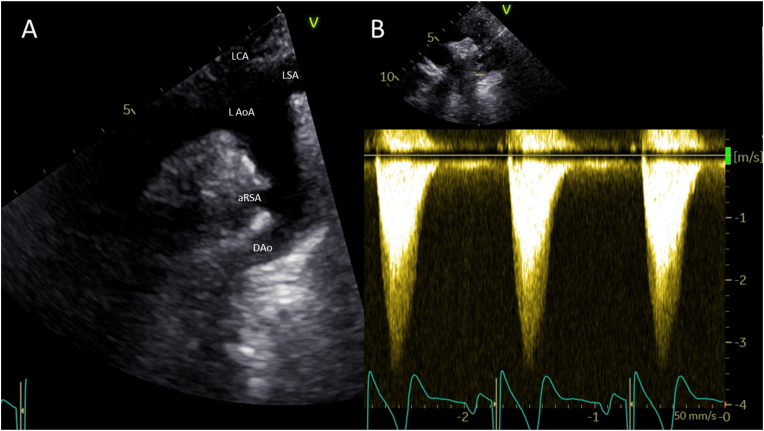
Figure 2: Transthoracic echocardiography of the aortic arch. A: 2 dimensional image of the arch (aRSA: anomalous right subclavian artery, DAo: descending aorta, L AoA: left-sided aortic arch, LCA: left carotid artery, LSA: left subclavian artery). B: Continuous Wave Doppler signal in descending aorta with peak forward flow rate of 3.5 m/s (gradient 49 mmHg) without diastolic run-off.
